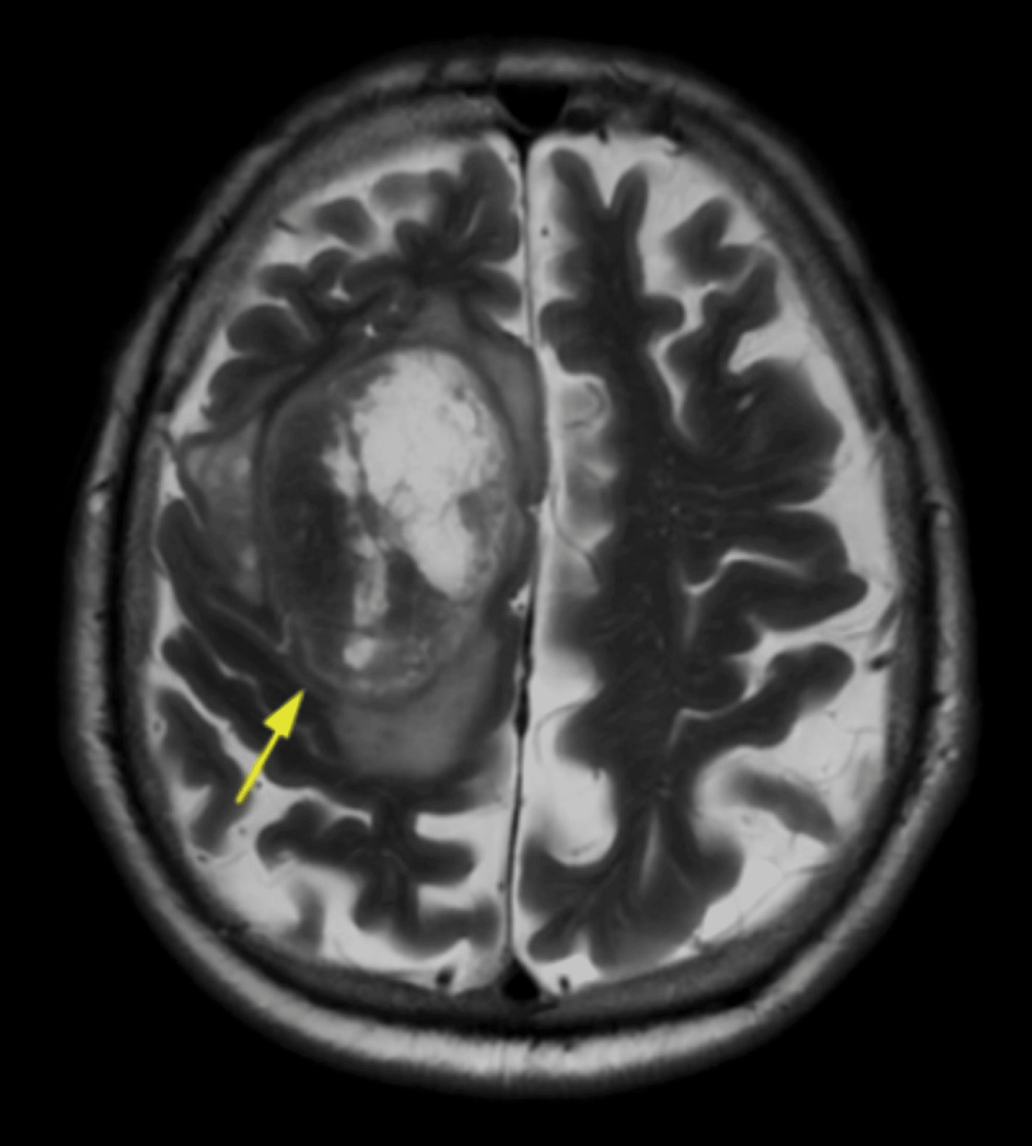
MRI of the brain showing heterogenous solid cystic mass in the right frontal lobe (arrow) MRI: magnetic resonance imaging
